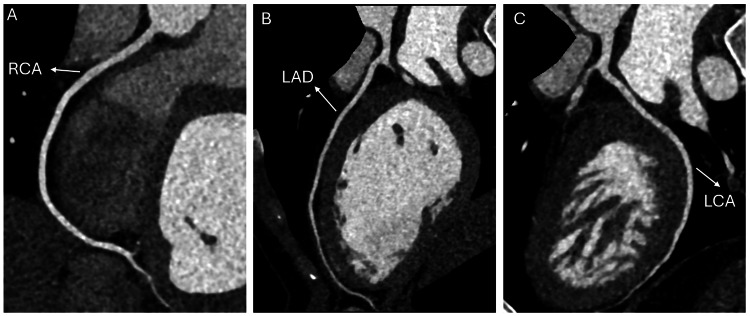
CT Cardiac angiogram coronary Normal origins and courses of right coronary artery (RCA) (A), left anterior descending artery (LAD) (B) and left circumflex artery (LCA) (C) with no stenosis or filling defects.
